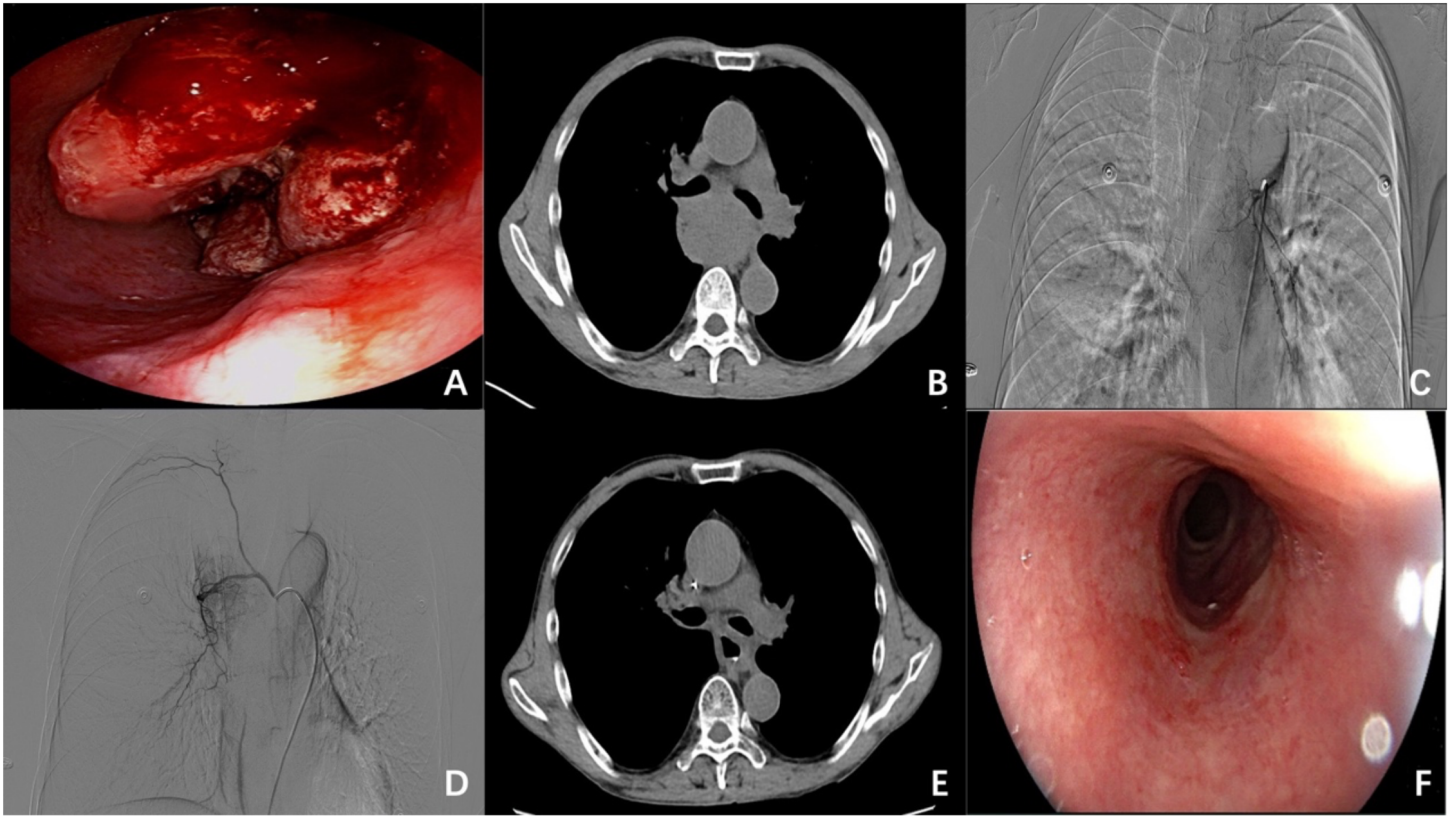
Imaging, endoscopic and DSA findings of Case 2: (A) Gastroscopy demonstrates a neoplasm causing significant luminal stenosis with active bleeding at 28–35 cm from the incisors. (B) Chest CT reveals marked wall thickening in the mid-esophagus. (C, D) DSA shows tumor blood supply from esophageal branches of bilateral bronchial arteries with prominent tumor vascularity. (E) Follow-up chest CT at 1-month post-combination therapy shows complete resolution of the primary tumor. (F) Post-treatment gastroscopy at 3 months displays radiation-induced mucosal changes without residual tumor.
